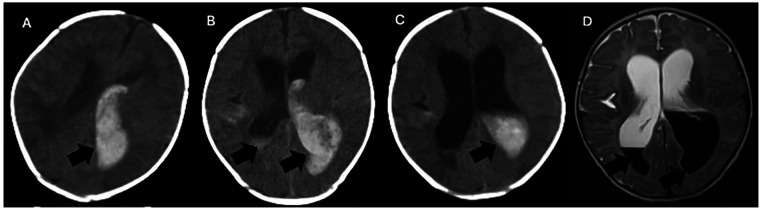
Head CT and MRI. (A–C) Serial non-contrast computed tomography (CT) scans of the brain. (A) Initial CT: A patchy hyperdensity, suggestive of acute hemorrhage, is seen in the left temporo-occipital lobe and bilateral lateral ventricles. (B) Follow-up CT: The intraventricular hyperdensity shows an interval increase in volume and extent compared to (A). (C) Subsequent CT: The previously noted hyperdensity demonstrates an interval decrease, consistent with partial resolution of the hemorrhage. (D) Corresponding brain magnetic resonance imaging (MRI). A focal lesion within the bilateral lateral ventricles exhibits short T1 and long T2 signal intensities. On the T2-FLAIR sequence, the lesion is hypointense, a signal characteristic consistent with residual methemoglobin.
